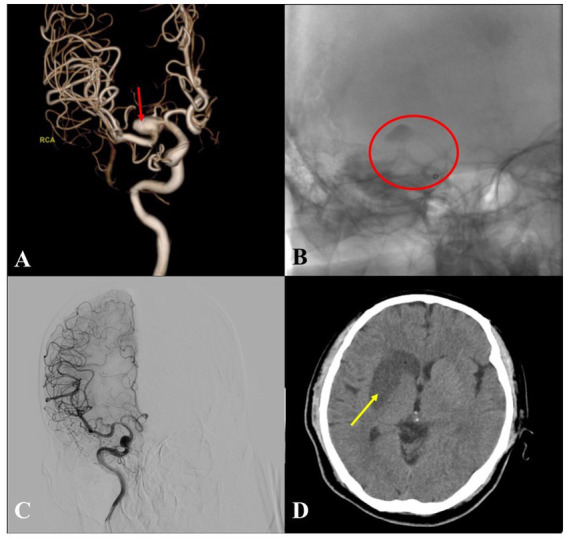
A case of postoperative cerebral infarction after PED implantation. (A) Intraoperative 3D angiography revealed a non-saccular IA in the M1 segment of the right middle cerebral artery (red arrow). (B) A 3.25 × 25 mm PED Flex was placed in the right C7–M1 segment, and marked remnant of contrast within the IA was observed (red circle). (C) On the second day after intervention, the patient developed weakness in the left side of the body. Follow-up DSA revealed that the IA had almost completely disappeared, and the right middle cerebral artery remained patent; immediately intravenous infusion therapy with Tirofiban was initiated. (D) A follow-up head CT scan on the third day after intervention revealed a cerebral infarction in the right basal ganglia region (yellow arrow).
